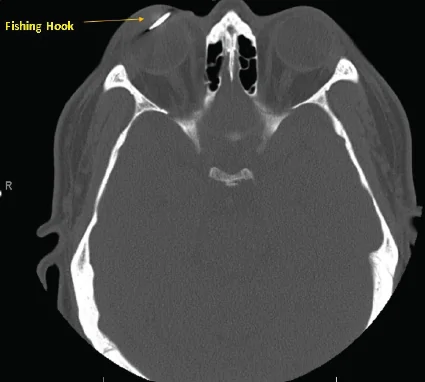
Computed tomography of the orbits, axial image of foreign body in anterior chamber of the right eye.
